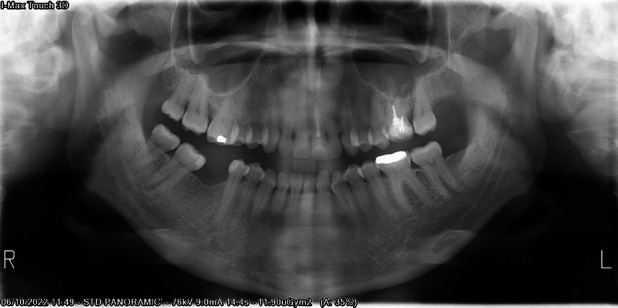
Panoramic radiography (performed after the extraction of tooth 28). No radiolucent images or increase in radiological density are observed in the posterior sector of the third mandibular quadrant.
